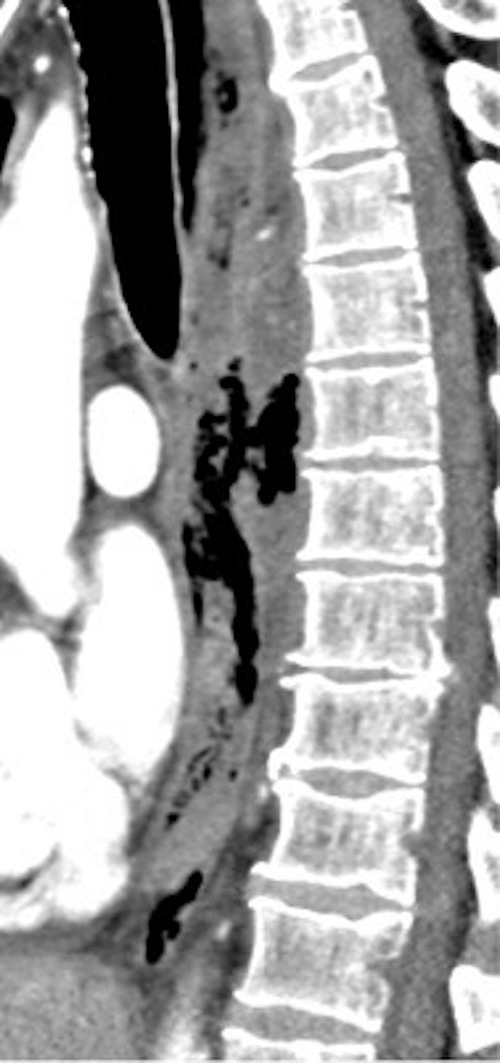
Sagittal CECT demonstrating a large amount of pneumomediastinum in the middle mediastinum with apparent discontinuity of the posterior esophageal wall. CECT, contrast-enhanced CT.
